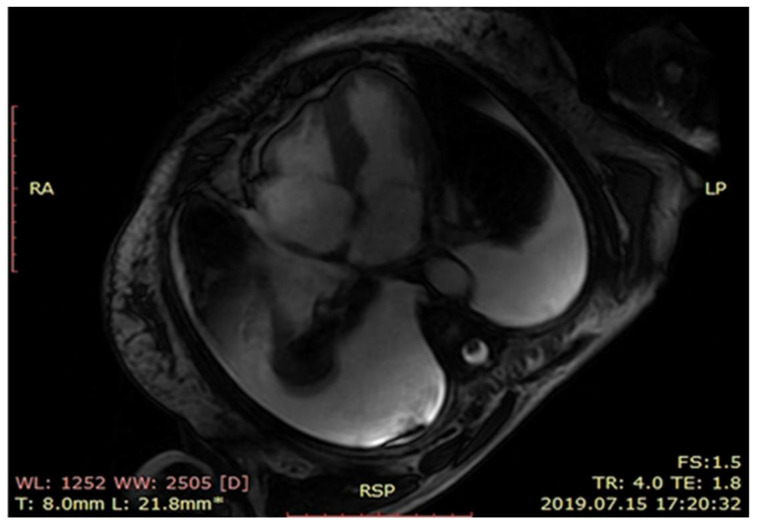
MRI scan showing left ventricle hypertrophy.
